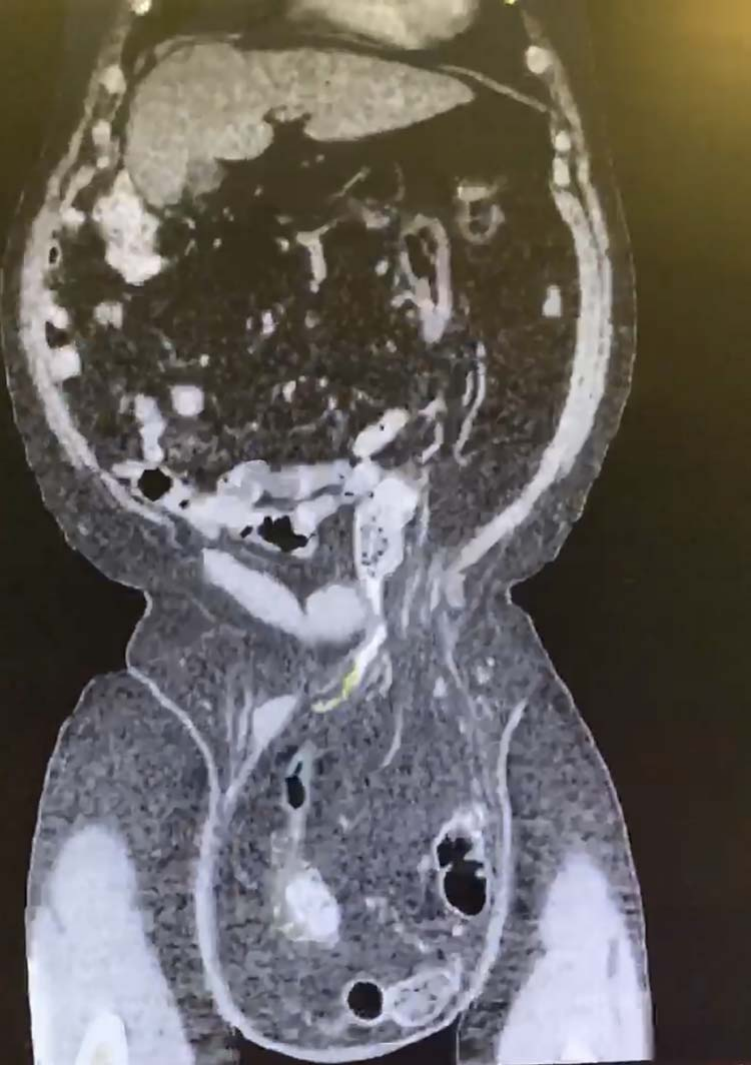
Coronal CT displaying sigmoid colon and accompanying abdominal contents traversing into the hernia sac.
